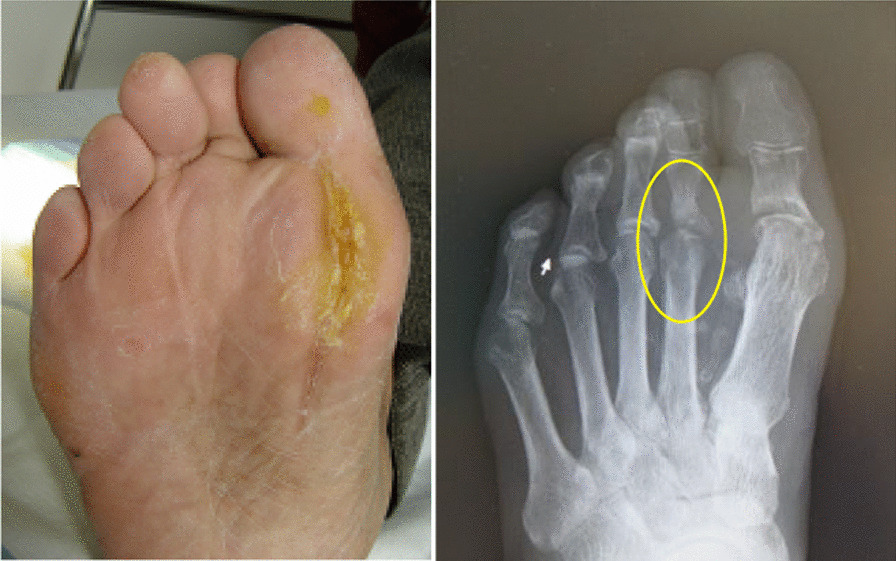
One year and five months later, the wound was healing and radiographs showed the regeneration of a joint-like structure in the metatarsophalangeal joint of the second toe. Yellow circle indicates the regeneration of a joint-like structure
